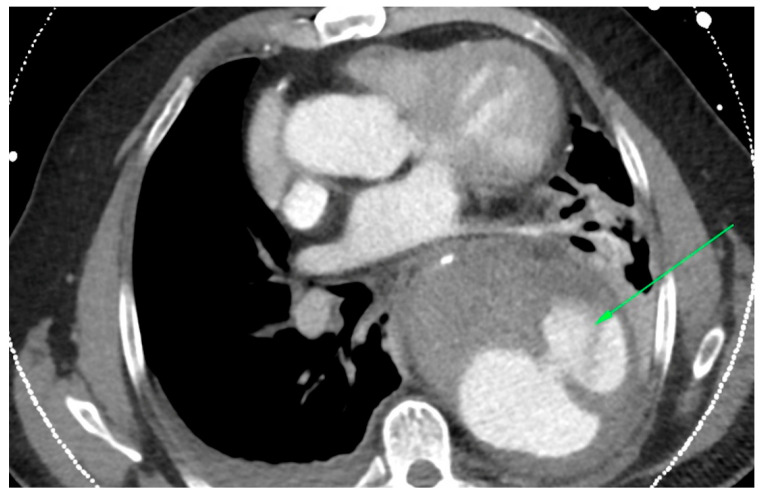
CT-scan of a thoracic aortic aneurysm rupture (green arrow).
